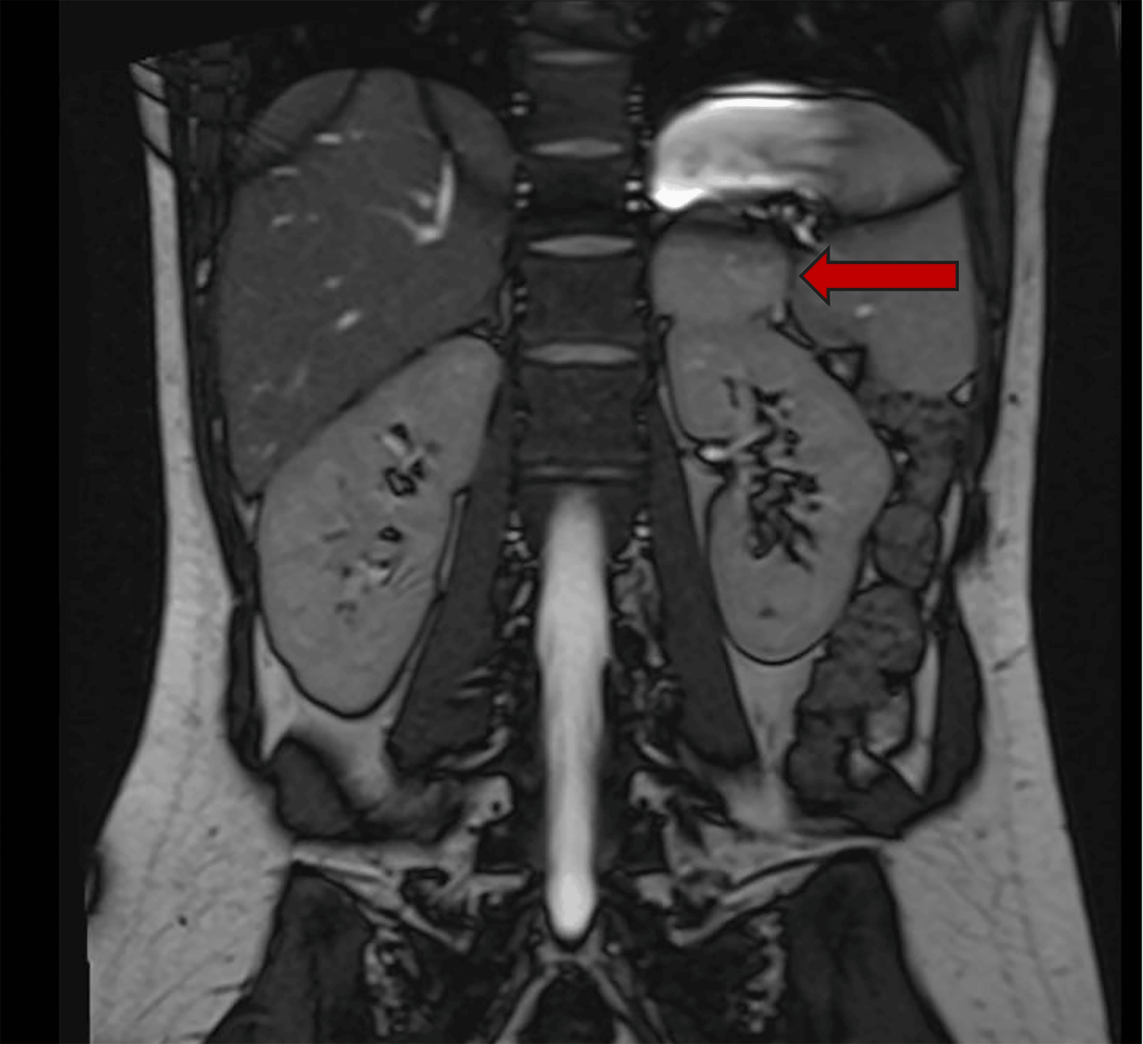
MRI of the adrenal gland The red arrow points to the left adrenal mass.
